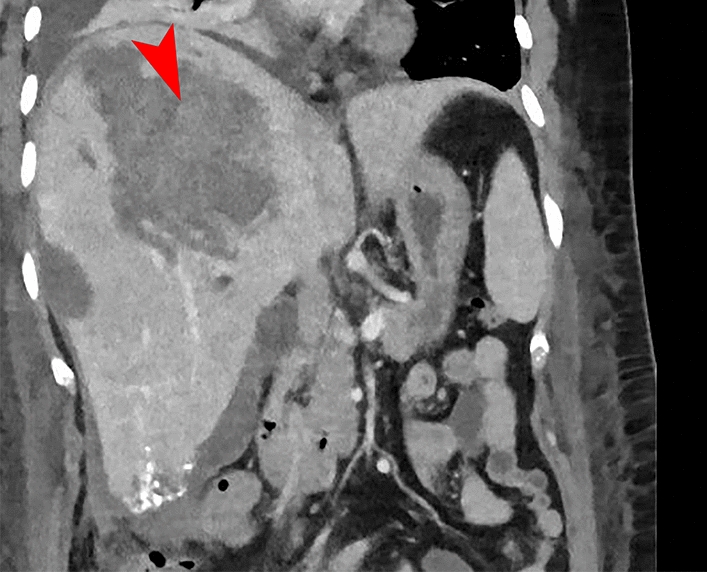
Abdominal computed tomography showing a recurrent/new bleed from the adenoma in segment 7 (marked with red arrow). Additionally, you can see the result of embolization with histoacryl of the adenoma in segment 5/6
